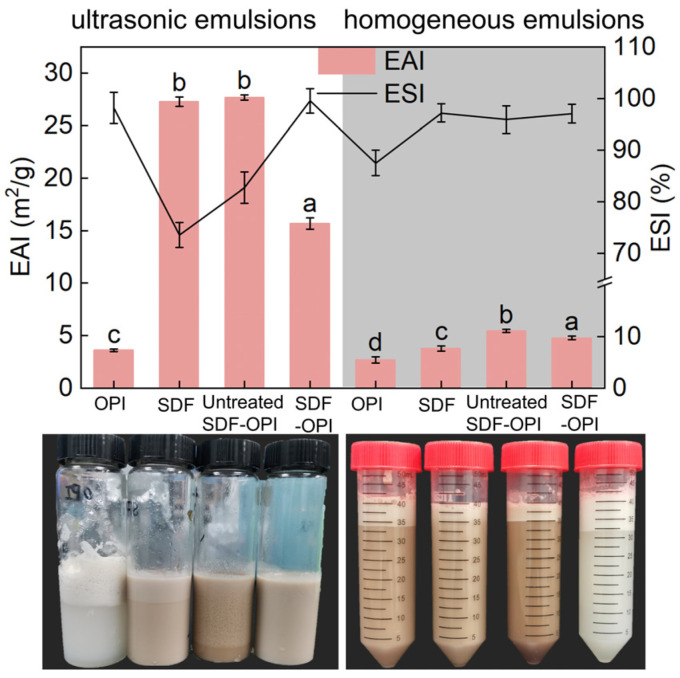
Emulsification (EAI) and emulsion stability (ESI) of ultrasonic emulsions (left) and homogeneous emulsions (right) of OPI, SDF, untreated SDF-OPI, and SDF-OPI complex. ^a,b,c,d^: Different lowercase letters indicate significant differences (*p* < 0.05).
